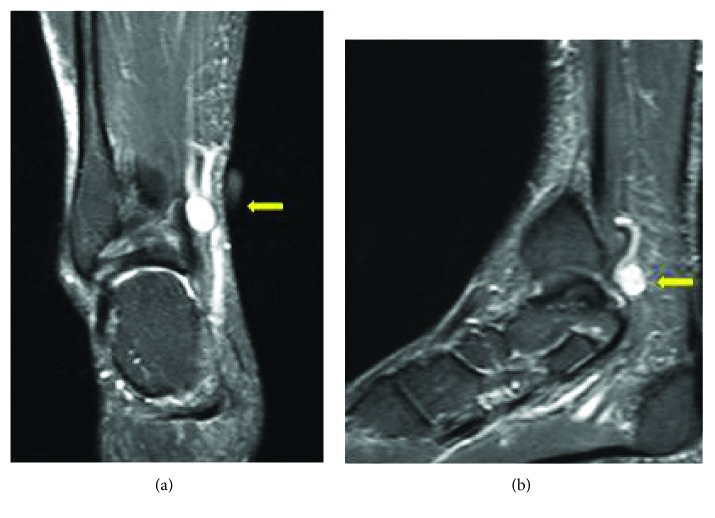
Well-defined hyperintense lesion (depicted by arrows) seen involving the posterior tibial nerve in T2-weighted MRI (a, b).
